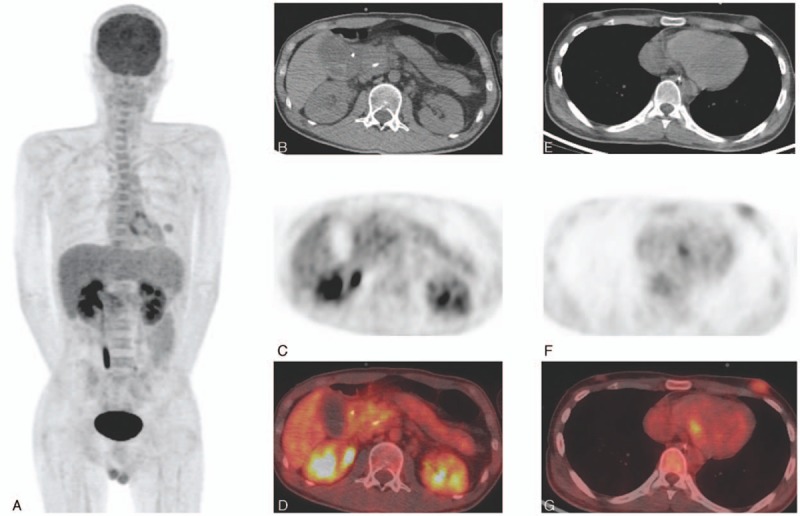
Imaging findings on ^18^F-fluorodexyglucose positron emission tomography/computed tomography. Three focal lesions (A) with moderate metabolic activity were observed in the pancreatic head ([B], computed tomography [CT]; [C], positron emission tomography [PET]; [D], fusion), in the skin in his left breast area ([E], CT; [F], PET; [G], fusion), and in the abdominal skin.
